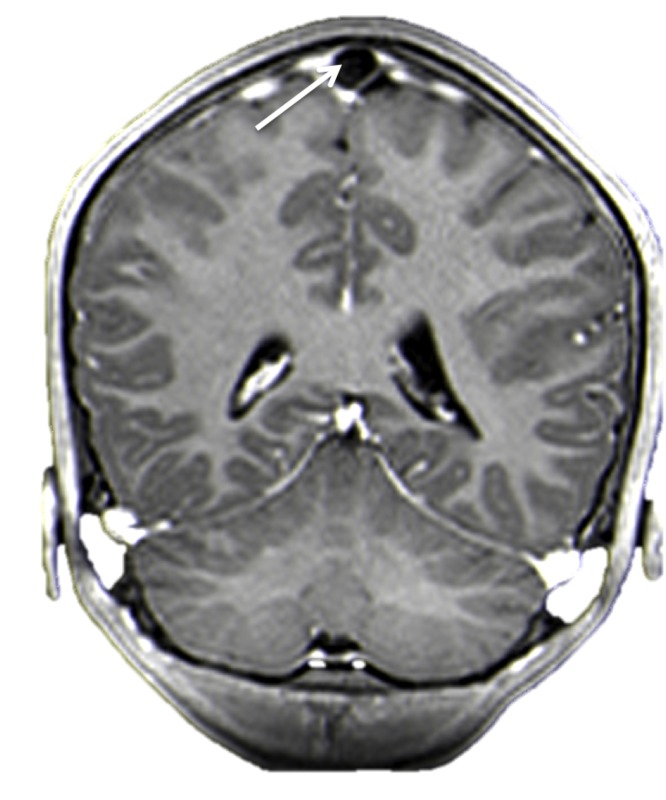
Contrasted coronal MRI of the head. Note the large hypodense area (arrow) within the superior sagittal sinus. This was diagnosed as a giant AG.
